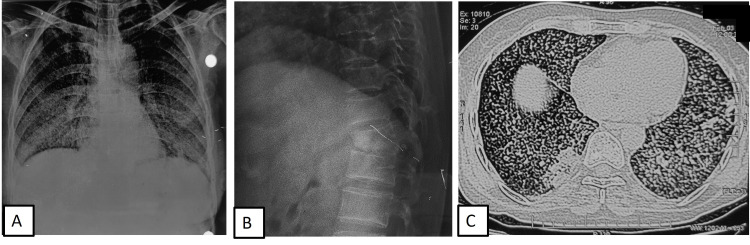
(A) Chest radiograph showing bilateral middle and lower zone nodules. (B) X-ray lateral view showing lower thoracic vertebra (D12) involvement. (C) Chest CT showing bilateral random nodules with focal consolidation in the right lower lobe.
